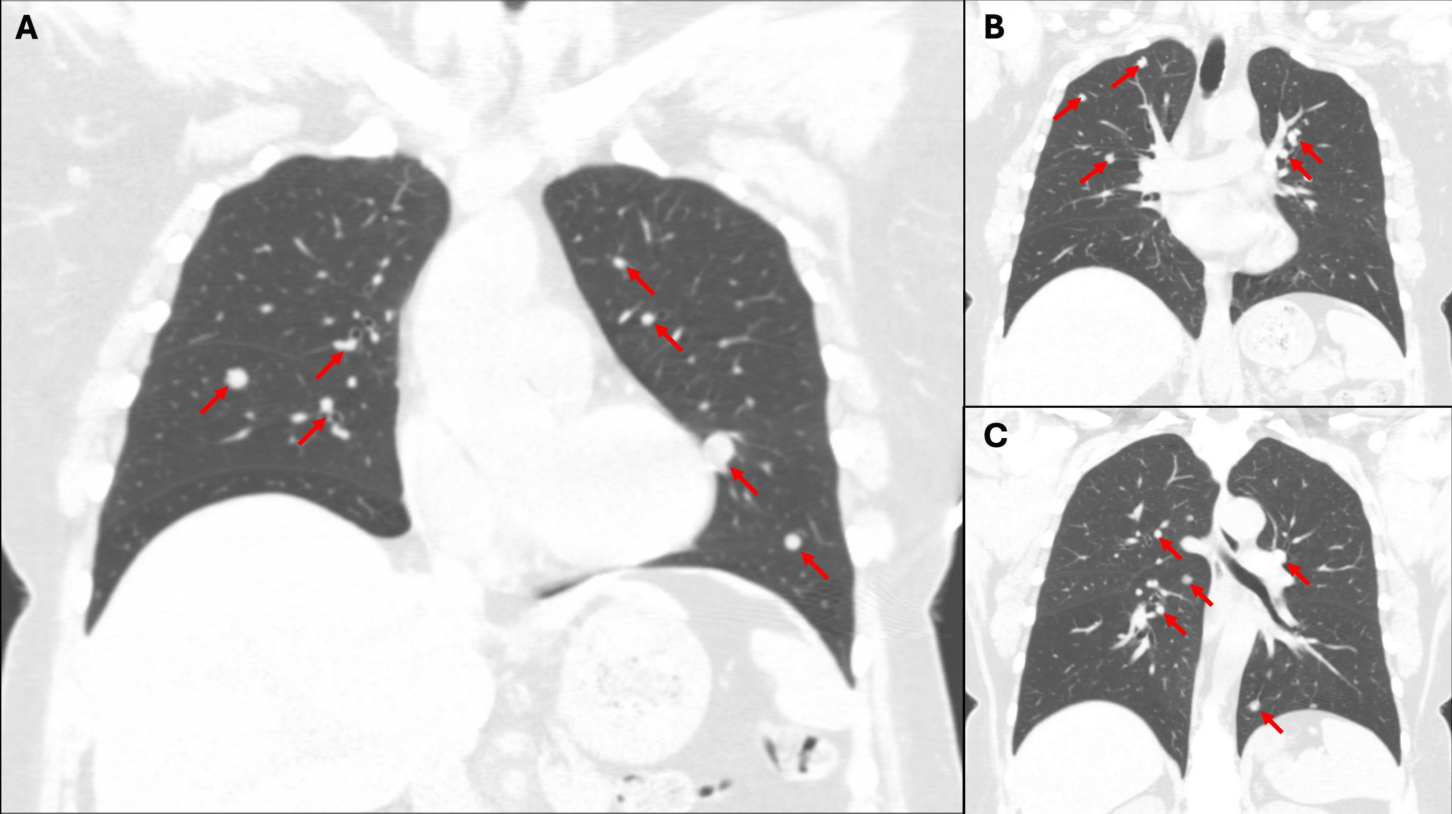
Computed tomography imaging demonstrating numerous bilateral pulmonary nodules. (a)-(c) Coronal reconstructions of the chest computed tomography. Representative pulmonary nodules are indicated using red arrows.
